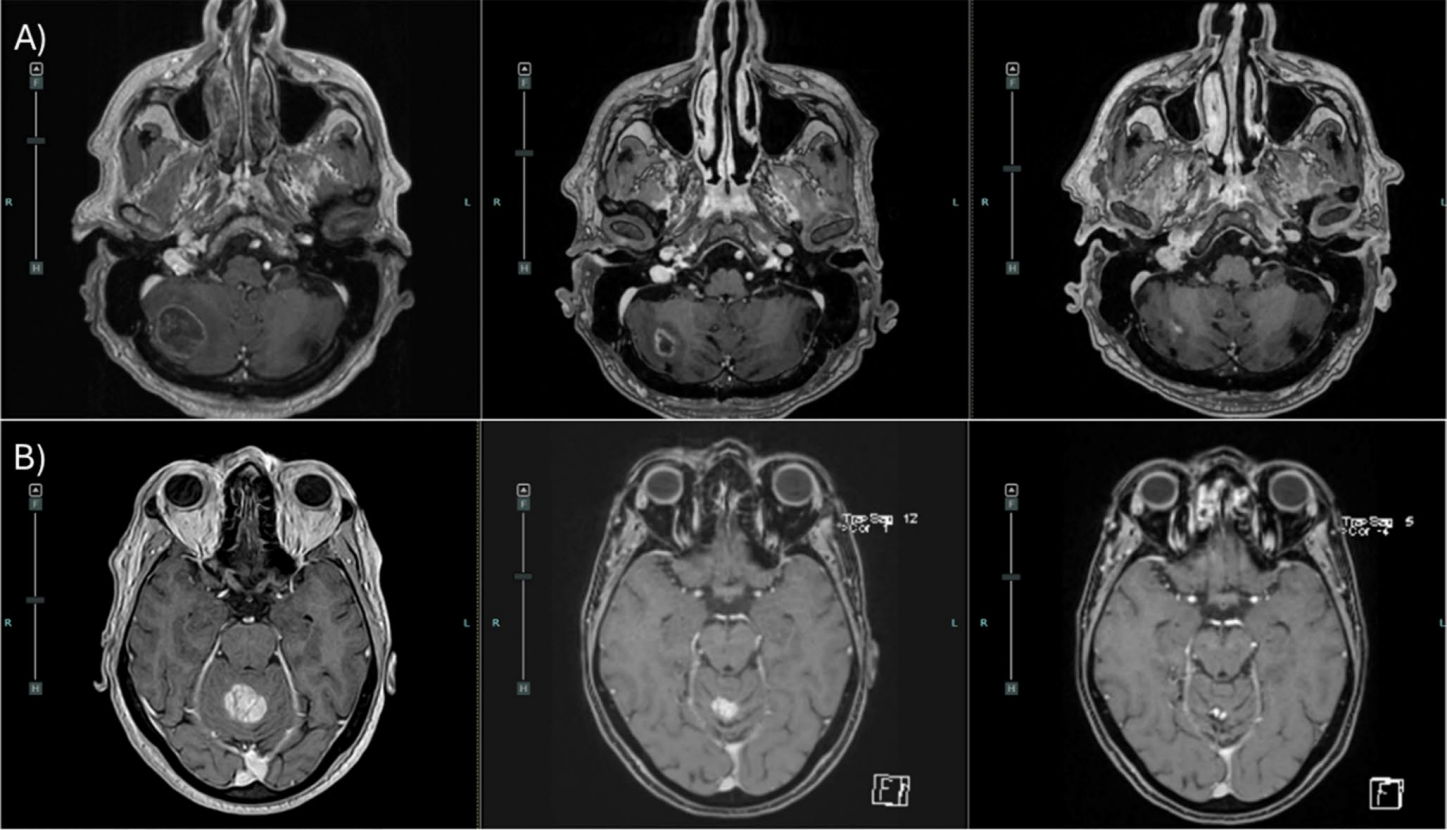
Axial post-contrast T1-weighted MRI scans showing cerebellar metastases and SRS treatment response. (A) A 75-year-old patient with a right cerebellar lesion (9.31 cm³) treated with single fraction SRS (20 Gy to the 80% isodose line). (B) A 58-year-old patient with a superior vermian lesion (6.3 cm³) treated with FSRS (28 Gy in 4 daily fractions to the 80% isodose line). Images are shown at baseline (left), 3 months (middle), and 6 months (right) post treatment
